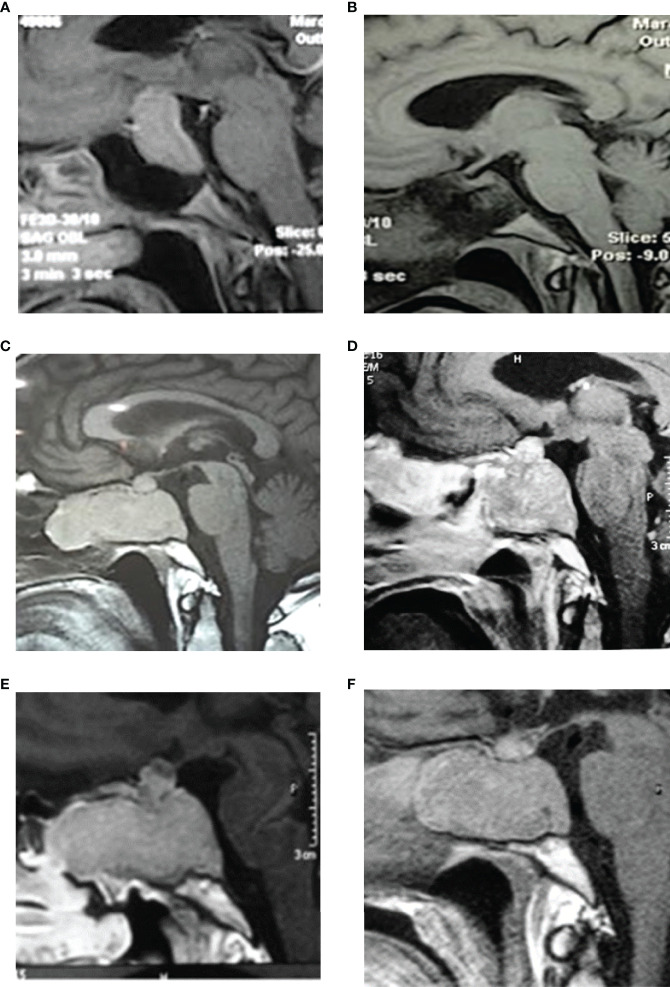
MRI images. (A) 2008-Preoperative pituitary MRI with gadolinium (sagittal view), evidencing a large pituitary mass with suprasellar extension and compression of the optic chiasm. (B) Postoperative MRI with gadolinium (sagittal view) showing complete tumor resection and decompression of the optic chiasm. (C) 2014-pituitary MRI (sagittal view) evidencing a tumor mass with extensive sphenoid and nasal invasion. (D) Postoperative MRI with gadolinium (sagittal image) showing partial resection with a remnant tumor in the nasal, sphenoid, and sellar region. (E) Control MRI in 2015 shows tumor regrowth in the sagittal image. (F) Postoperative MRI (sagittal view) showing less remnant tumor mass than in 2015. Tumor remains stable after radiotherapy and both cabergoline and ketoconazole treatment until 2021.
